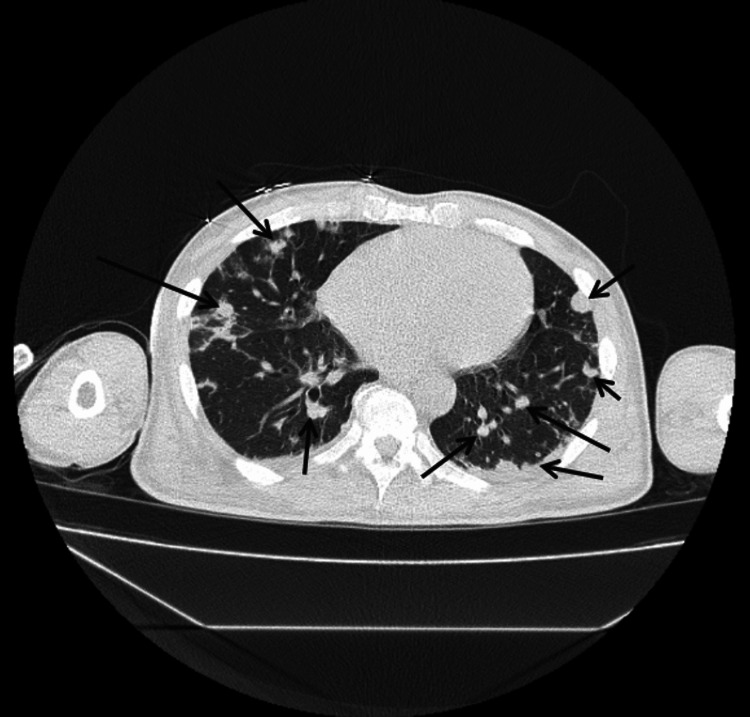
CT chest axial view without contrast showing the lower parts of both lungs. The arrows show multiple, bilateral diffuse airspace cavitations with air bronchograms in some cavities, consistent with septic pulmonary emboli.
